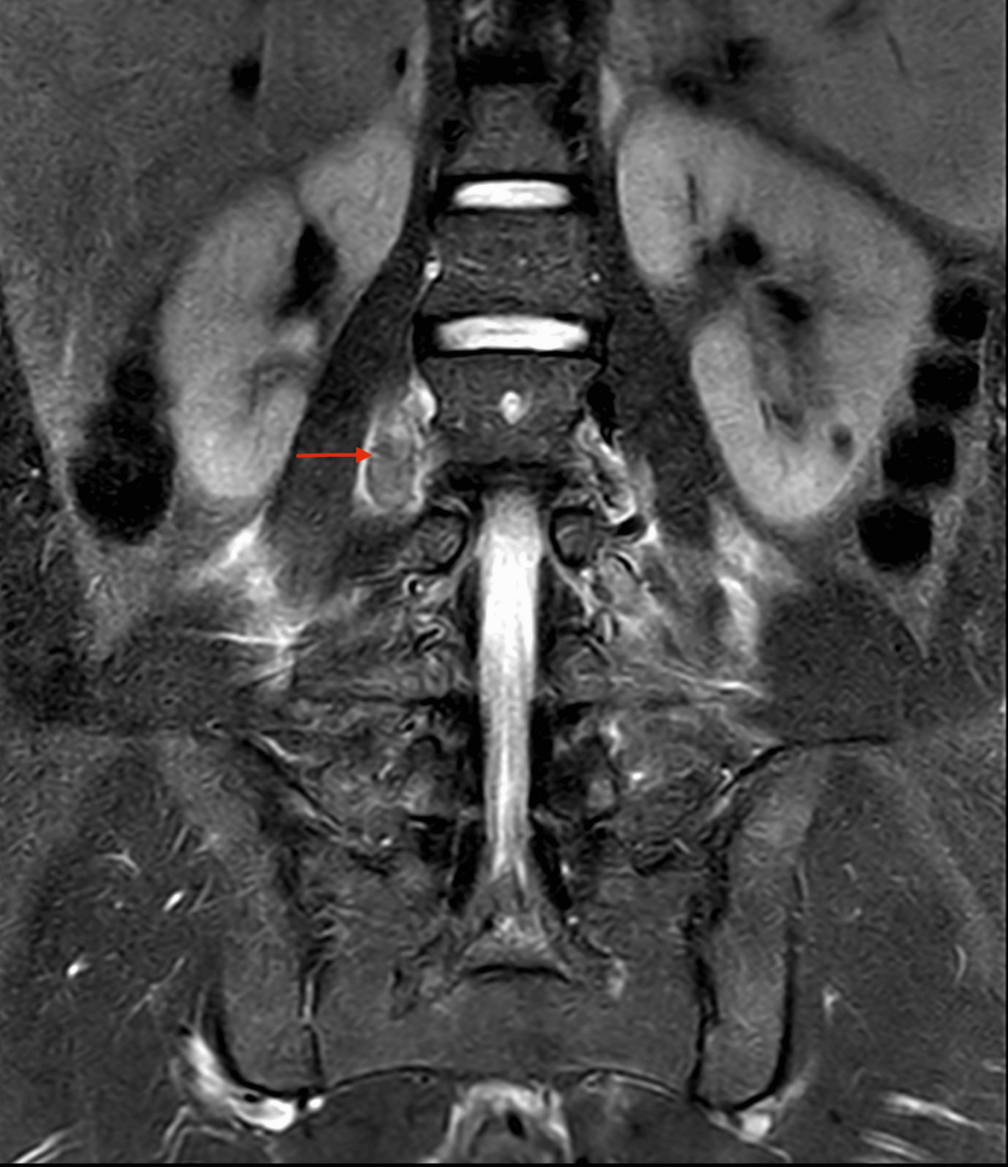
Lumbar spine MRI showed the presence of prominent collateral veins due to IVC atresia IVC: inferior vena cava
